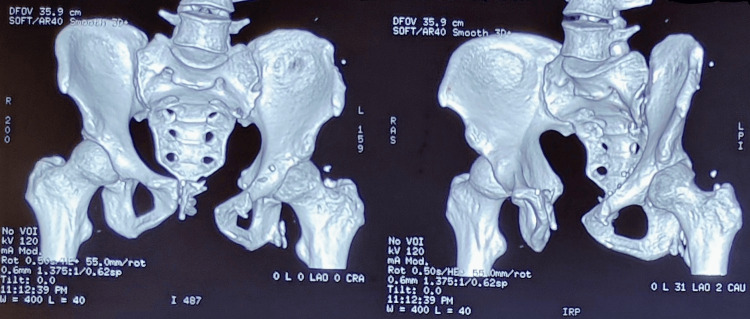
Three-dimensional computed tomography reconstruction of the pelvis revealing pubic diastasis
